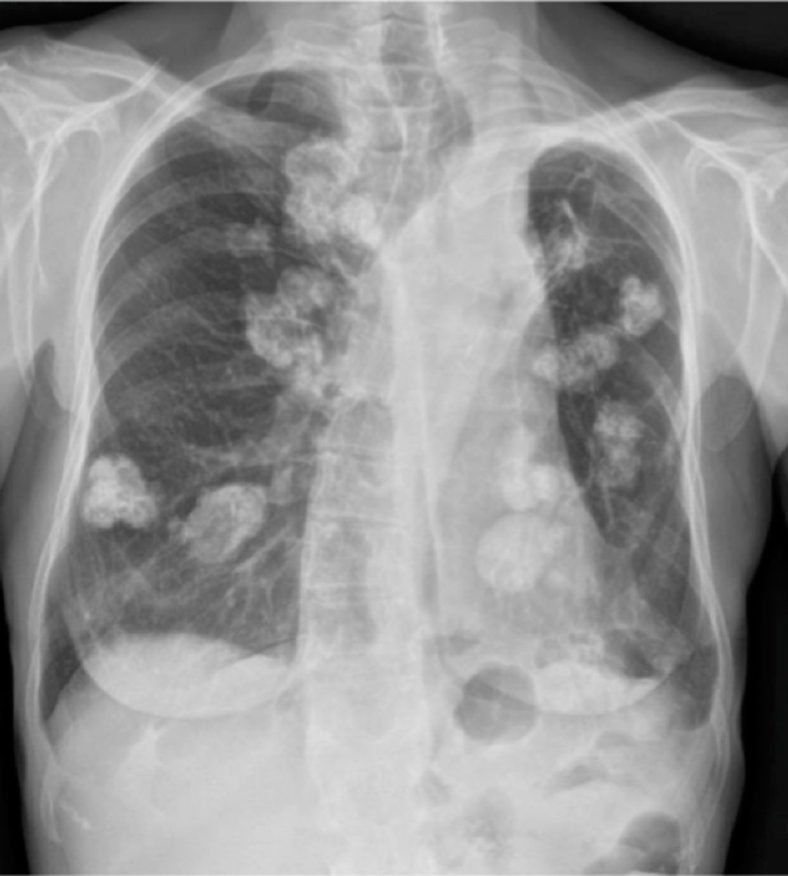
Preoperative radiograph of the chest showing the presence of multiple bilateral pulmonary chondromas.
